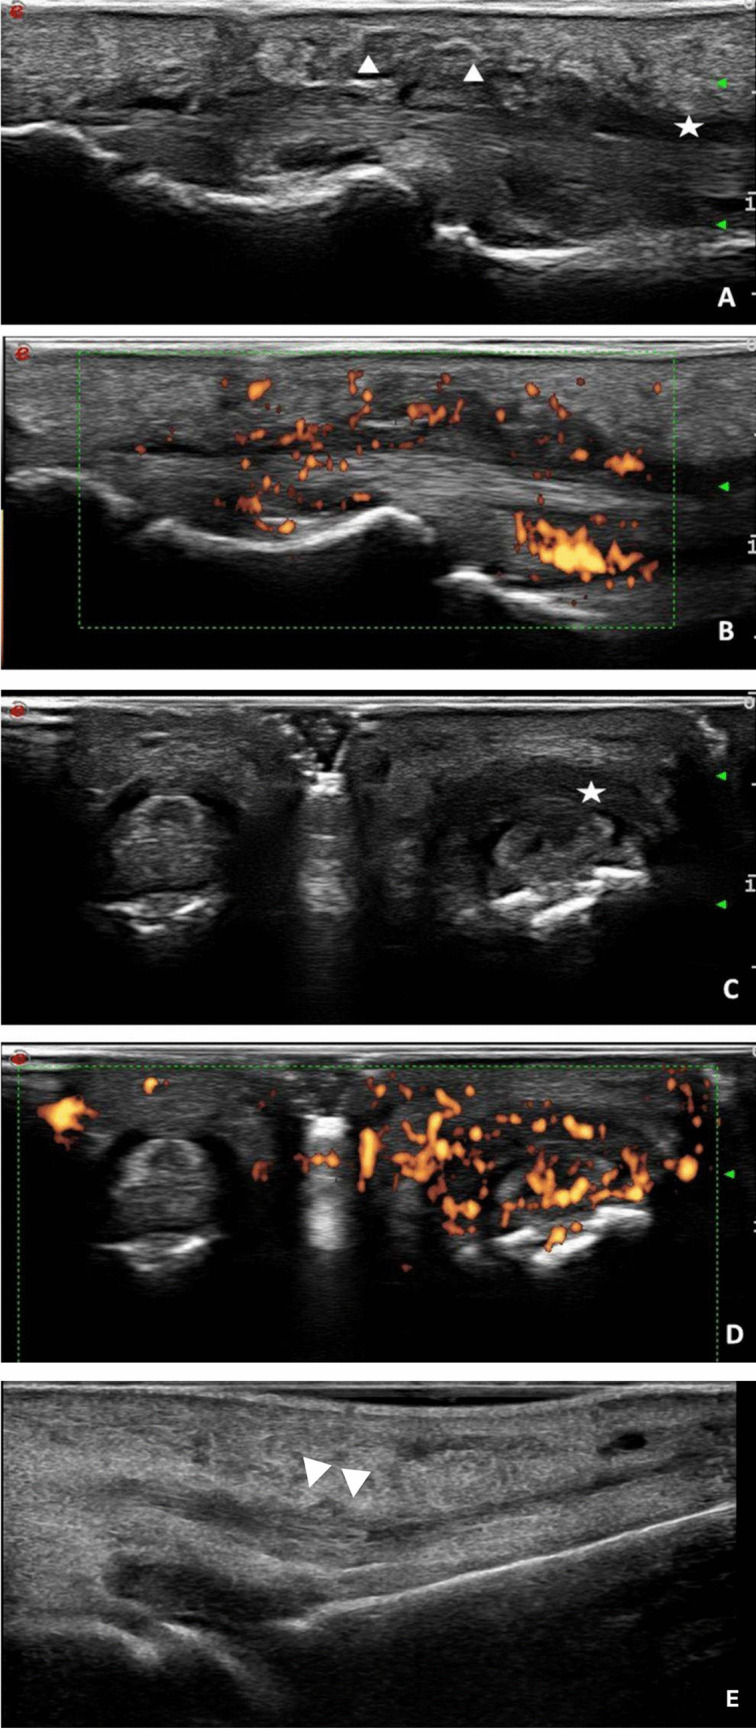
2.1 Longitudinal ultrasound views of toe dactylitis. A: Flexor tenosynovitis (white asterisk) with soft tissue oedema (white arrowheads). B: Presence of power Doppler signal (PDS) around the tendon fibers and in subdermal tissue. 2.2 Transverse ultrasound views of toe dactylitis. Comparison between a toe affected by dactylitis (right) and a healthy toe (left). C: The affected toe shows flexor tenosynovitis (white asterisk). D: In the affected toe there is PDS around the tendon fibers and minimally at the level of the subdermal tissue. 2.3. E: Longitudinal ultrasound view of toe dactylitis. Peritendinitis is shown by white arrowheads
